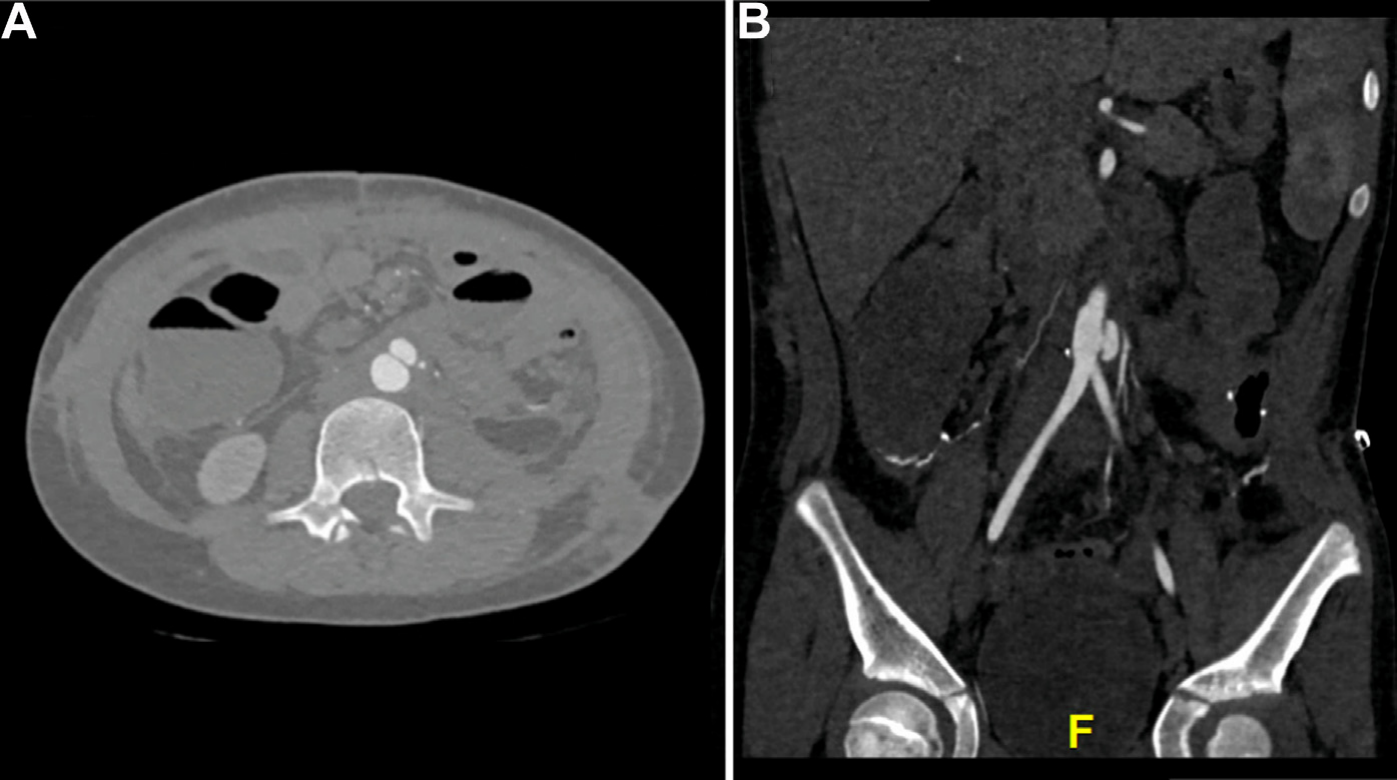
A, Computed tomography (CT) angiogram obtained 1 month after injury showing a rapidly degenerating aortic pseudoaneurysm. B, CT angiogram in coronal view with angle adjustment to demonstrate the location of the pseudoaneurysm in relation to the aortic bifurcation.
